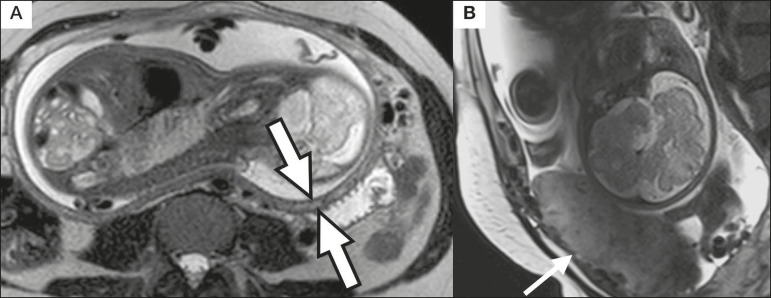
A: Axial T2-weighted MRI sequence showing a normal trilaminar appearance of the myometrium, represented by the middle layer with high signal intensity whereas the outer and inner layers have low signal intensity (arrows). B: Sagittal T2-weighted sequence showing myometrial thinning with indistinct layers (arrow).
